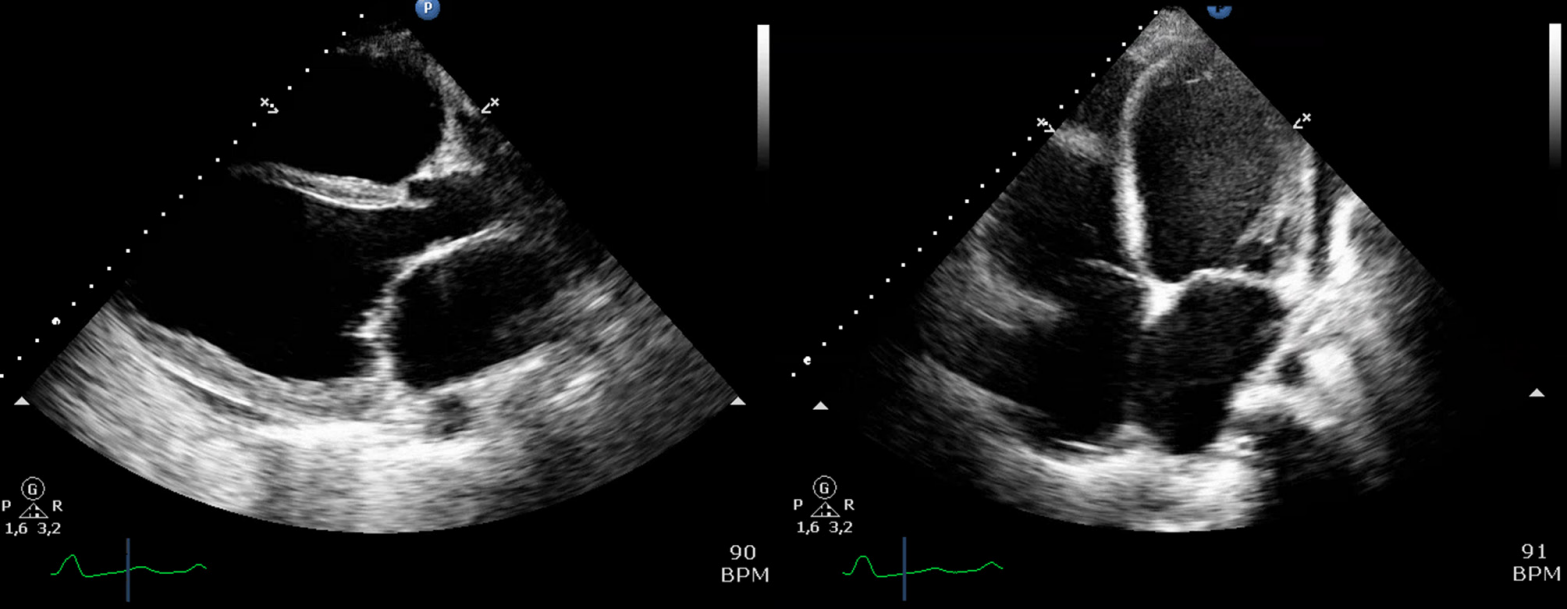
(A) Parasternal long axis on echocardiogram of patient B at diagnosis. The LVEDD was 76 mm. (B) Four-chamber apical view of patient B at diagnosis. Biventricular dilatation with severely impaired right and left ventricular function. Severe mitral regurgitation. BPM, beats per minute; LVEDD, left ventricular end diastolic diameter.
